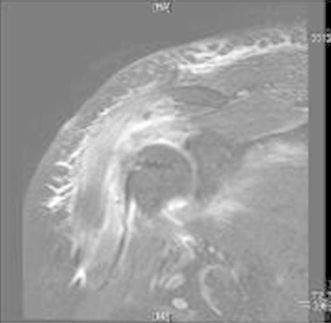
MRI appearances of the suture anchor and final tenodesis
